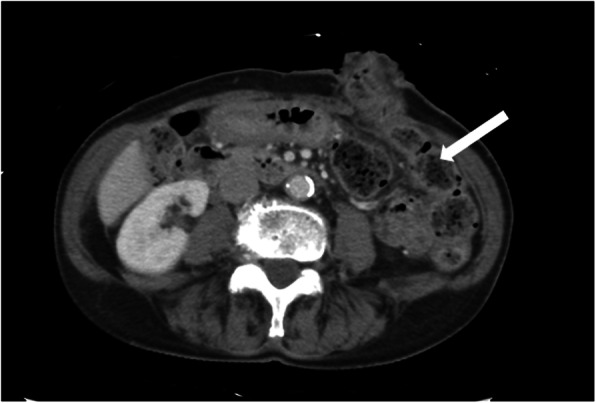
CT scan shows the lifted sigmoid colon fixed to the lateral abdominal wall
